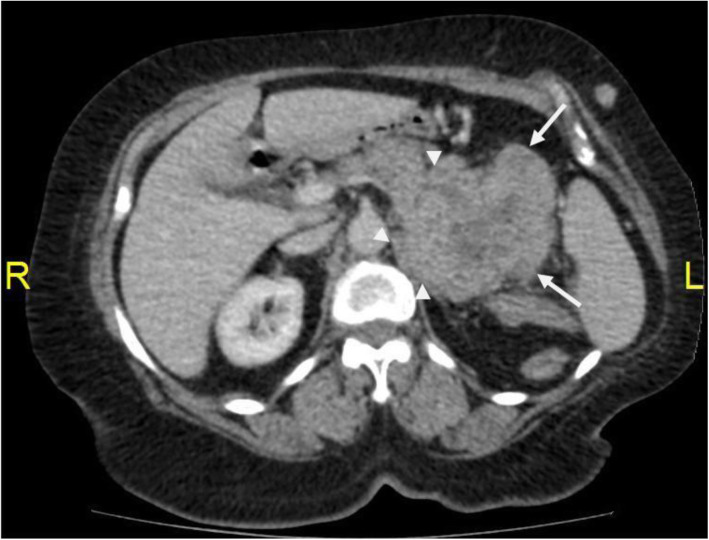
Contrast-enhanced CT scan of the abdomen showing large ill-defined soft tissue mass arising from the body and lateral limb of left suprarenal gland (arrows), and the mass exhibits heterogeneous postcontrast enhancement with areas of cystic degeneration, and it was seen inseparable from the body and tail of the pancreas as well as the left diaphragmatic crus (arrow heads)
